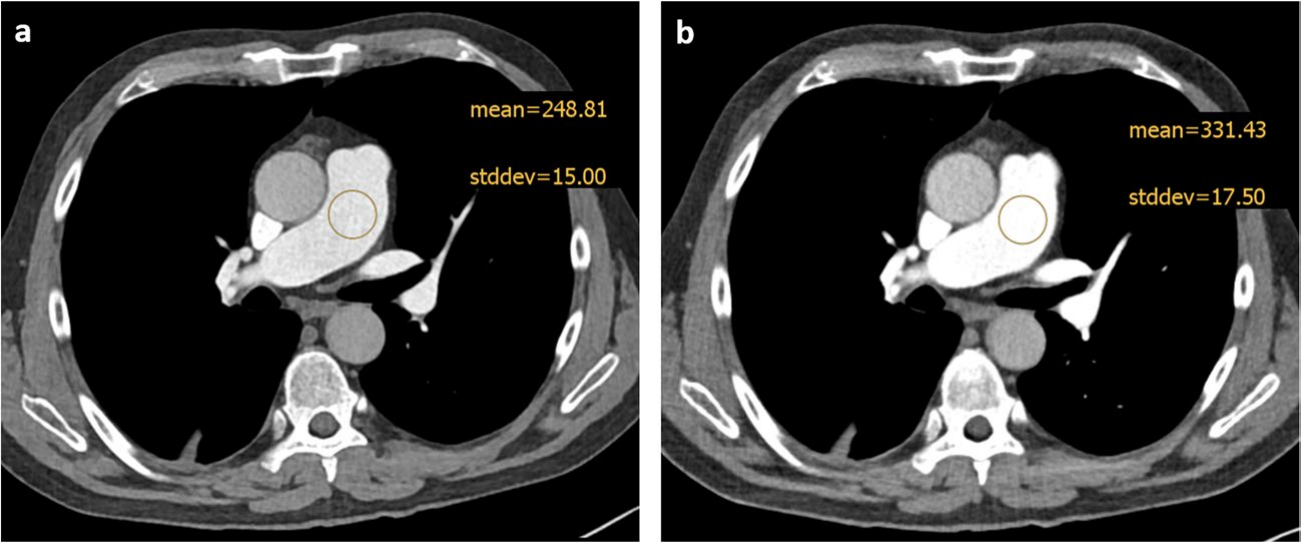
PCD-CT “T3D” (a) *vs*. 60-keV images (b): compared to polychromatic low-energy threshold 120 kV images—“T3D” (a), 60-keV images (b) provide significantly higher iodine contrast (mean) at similar noise (stddev) levels. *PCD-CT*, photon-counting detector CT
